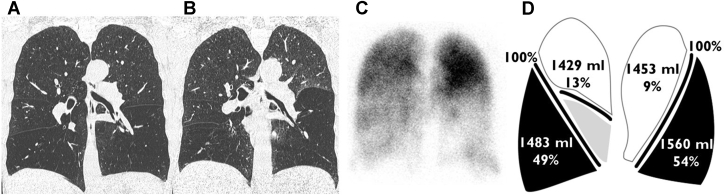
A-D, Case example of a suitable patient with COPD for bronchoscopic lung volume reduction with the use of one-way endobronchial valves. A, Inspiration chest CT scan shows lower lobe predominant heterogeneous emphysema; B, expiration chest CT scan shows predominant air trapping in the left lower lobe; C, perfusion scan confirms the left lower lobe target by absence of perfusion; D, quantitative CT analysis shows percentage of emphysematous destruction (at −950 Hounsfield Units), lobar volumes, and fissure integrity.
